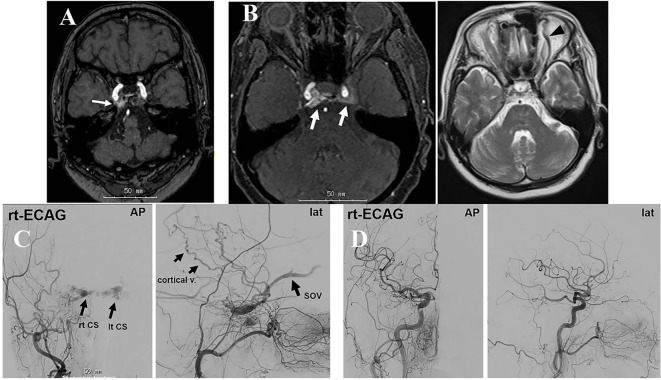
Case 3: (A) MRI obtained 2 months after the initial pain demonstrating an abnormal intensity in the right CS (arrow). (B) MRI/MRA obtained 10 months after onset showing abnormal blood flow in the bilateral CS (arrows) and dilatation of the left SOV (arrowhead). (C) Angiography showing abnormal blood flow in the right CS and SOV. The refluxed blood flows to the left CS via the intercavernous sinus and further to the left SOV. (D) Post-embolization angiography showing the disappearance of the abnormal blood flow.
